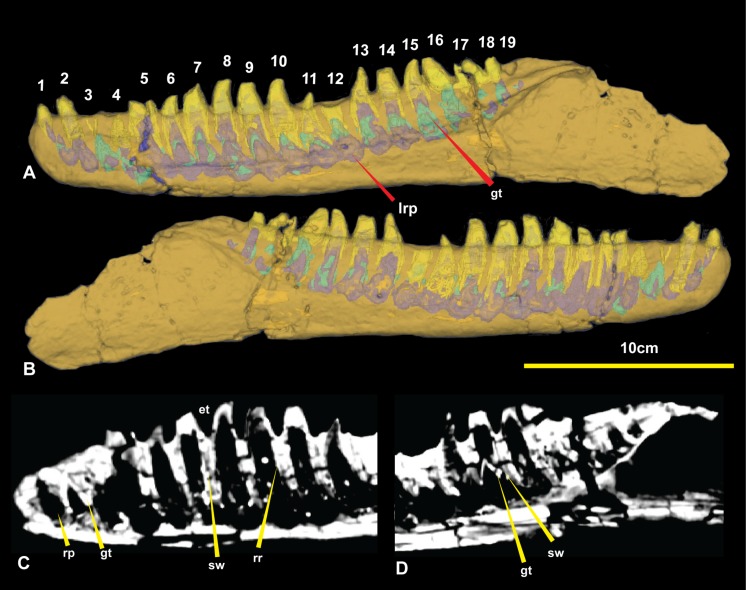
Computed tomography of right dentary. (A) Mimics render of lingual denary and internal structure; (B) Mimics render of labial dentary and internal structure; (C) CT scan of distal portion of dentary; (D) CT scan of proximal end of dentary. Abbreviations: gt, germ tooth; lrp, lingual resorption pit; rp, resorption pit; sw, socket wall; rr, residual root. Scale bar = 10 cm.
